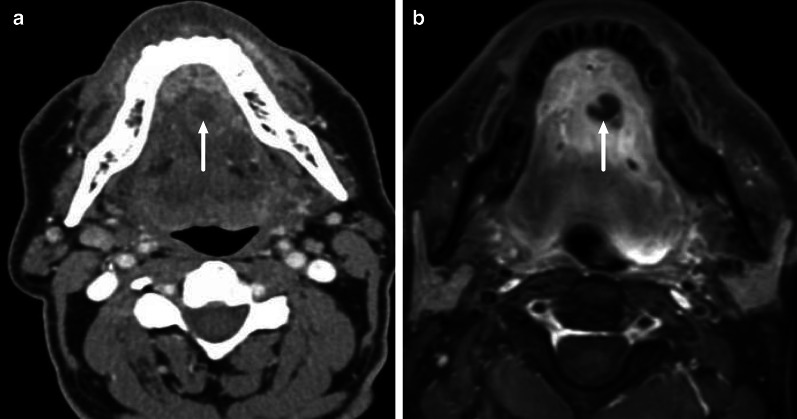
The CT diagnosis of an abscess may be difficult. Images show an abscess in the floor of the mouth on axial contrast-enhanced CT (a), and on axial T1-weighted fat-suppressed MRI after gadolinium (b) from the same patient imaged within 24 h with both modalities. Subtle central hypodensity and faint rim enhancement can be seen on CT (a); this was, however, misinterpreted as cellulitis. A fluid collection is clearly defined within abnormal tissue enhancement on MRI (b), and diffusion-weighed imaging (DWI, not shown here) suggested purulence, which was confirmed by surgery
